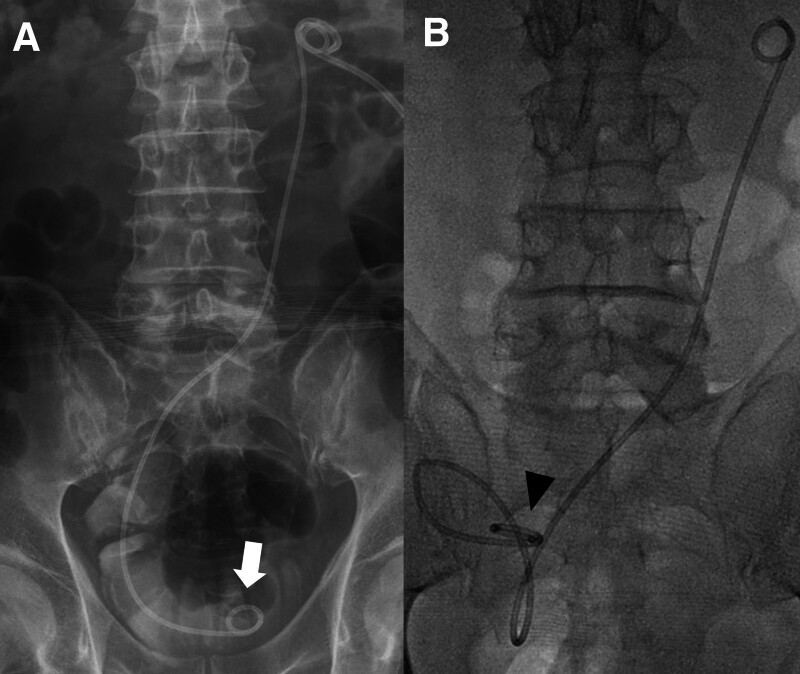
Seventy-seven-yr-old man with bladder cancer and undergone radical cystectomy and orthotopic neobladder. (A) Abdomen X-ray obtained just after placement of InLay Optima and showed proper located distal tip (arrow) of double-J ureteral stent (DJUS). (B) In 2 wk after placement of DJUS, abdomen X-ray showed proximal migration of the distal tip (arrowhead) of DJUS near ureteroileal anastomosis site.
